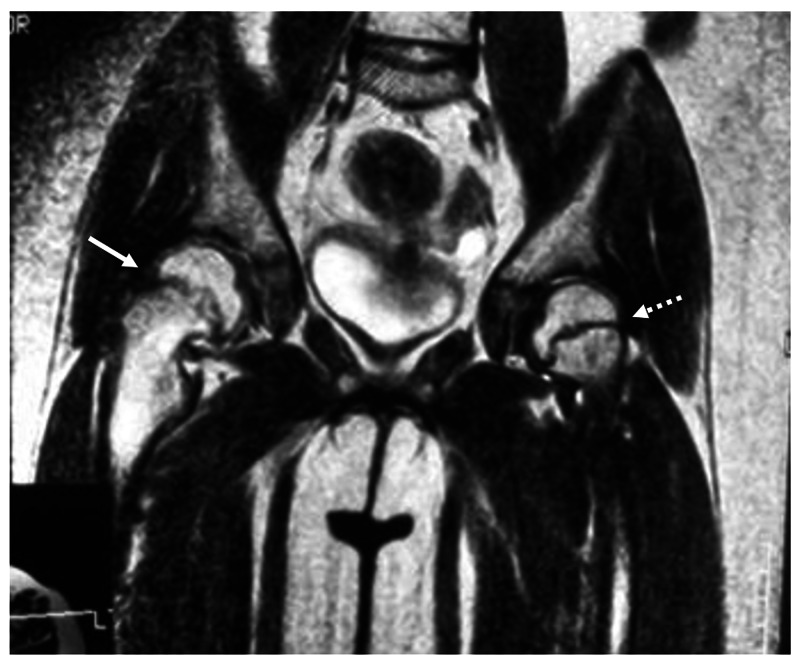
Preoperative MRI T2 image. A coronal MRI image shows bone marrow edema surrounding the right physis (arrow), but there were no findings suggesting pre-slip on the left (arrow of the thick dotted line).
